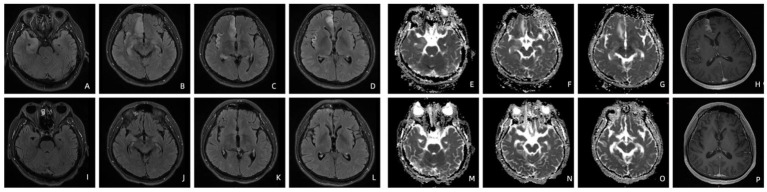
T2-FLAIR imaging of patient 1 before (A–D) and four months after treatment (I–L). The ADC (E–G) and contrast-enhanced T1-weighted imaging (H) of patient 1 before and four months after treatment (M–P).
